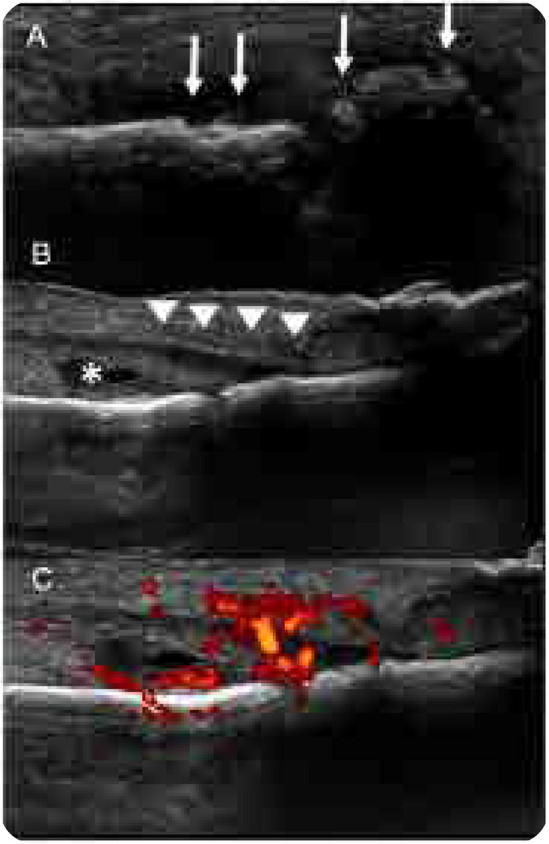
(left). Ultrasonography of the hallux revealing enthesitis characterised by hyperostosis of the proximal and distal phalanges (arrows in A), and increased thickness and hypoechogenicity of the extensor hallucis longus (arrowheads in B) associated with peritendinous positive Doppler findings adjacent to the tendon’s insertion at the base of the distal phalanx (C). Synovitis of the interphalangeal joint of the hallux (asterisk in B) is also visible.
